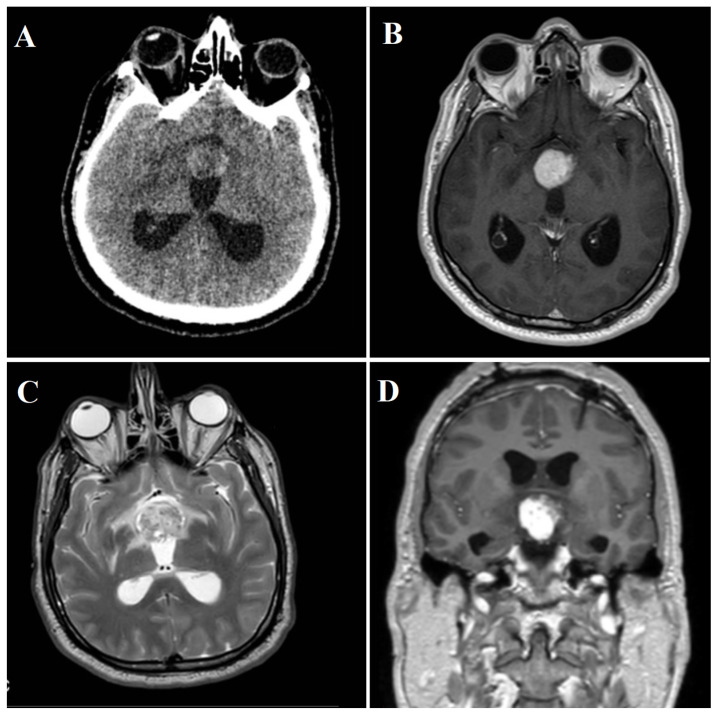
(A) Initial brain CT-scan showing a heterogeneous spontaneous hyperdense hypothalamic lesion with associated hydrocephalus. (B) Axial MRI appearance of the lesion, with intense gadolinium enhancement. (C) Inhomogeneous hyper-intensity on MRI T2-weighted images. (D) Coronal MRI appearance of the lesion, with intense gadolinium enhancement.
